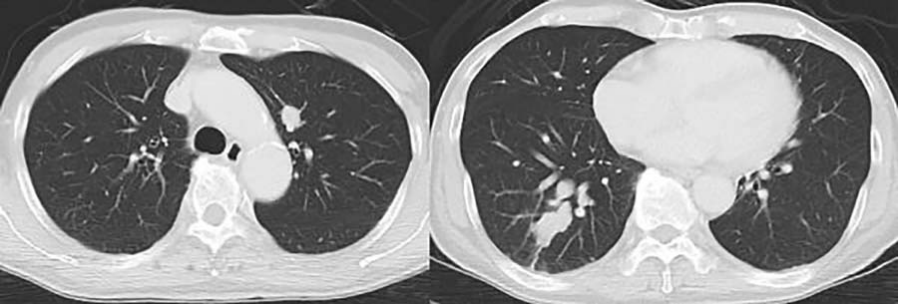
Computed tomography also showed irregular lung masses.
